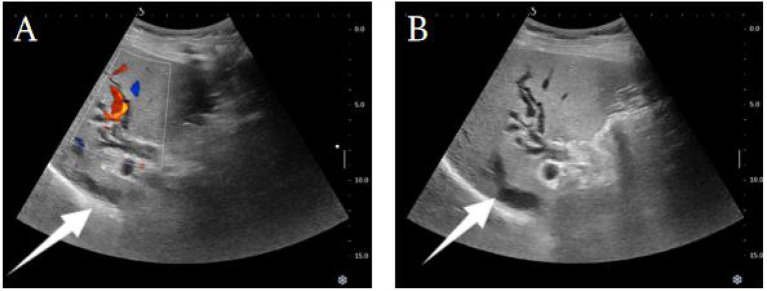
Upper abdominal ultrasound images. (A) Focal lesion in the pancreatic head region with dilatation of intra- and extra-hepatic bile ducts. Color Doppler imaging shows abnormal blood flow around the mass. (B) Focal lesion in the pancreatic head region with significant bile duct dilatation. No obvious abnormal blood flow around the mass is seen.
